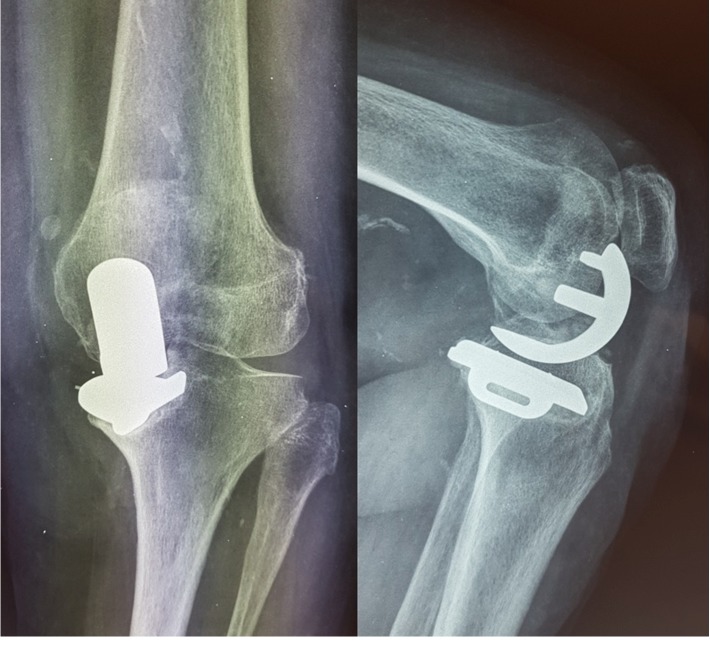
Anteroposterior and lateral X-ray views during initial evaluation. Mal-positioning of the unicompartmental knee arthroplasty with varus deformity is observed.
